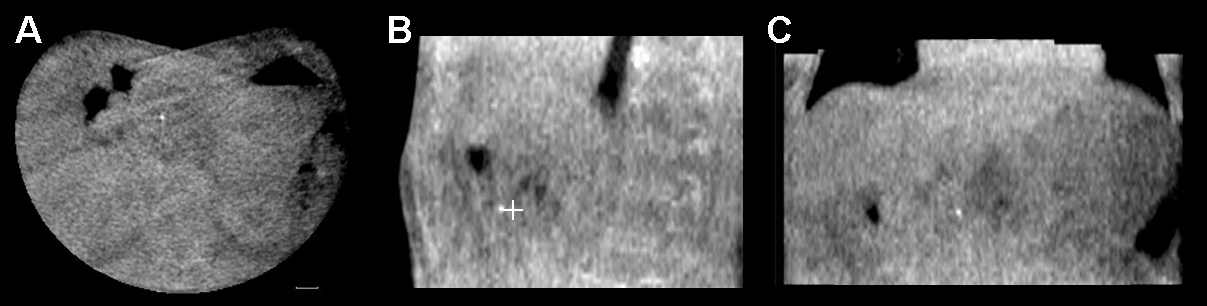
Axial (a), sagittal (b), and coronal (c) views of MV‐CBCT for one treatment session in patient #6. The fiducial appeared as a single blurred point. The position of the fiducial in the first MV‐CBCT is also shown (symbol ‘+’) in the sagittal section.
